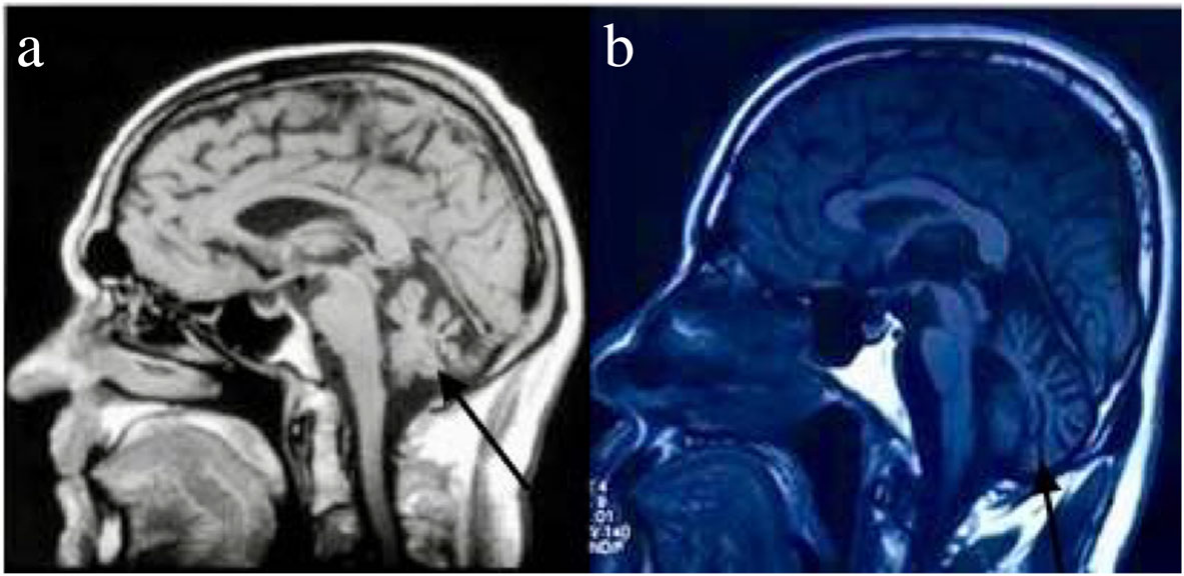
Neuroradiological examination by magnetic resonance imaging (MRI): (a) mid-sagittal MRI (T1 weighted image, T1WI) of the brain of patient II-5 (male proband; 49 years old at the time of MRI, 21 years after disease onset; and (b) mid-sagittal MRI (T1WI) of the brain of II-7, male sibling of proband; 48 years old at the time of MRI, 22 years after disease onset). The arrows indicate cerebellar atrophy
